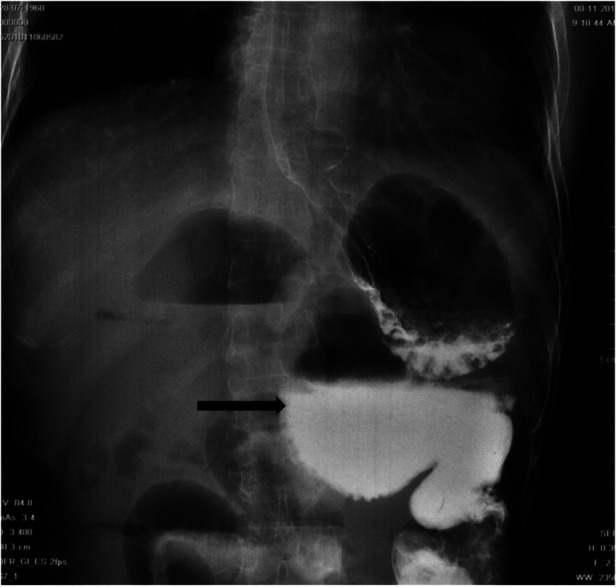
X-ray gastrointestinal radiography image: Ten minutes after taking barium, a large amount of contrast medium remained in the dilated duodenal cavity. The blind end of the duodenum filled with contrast medium was indicated by the black arrow.
